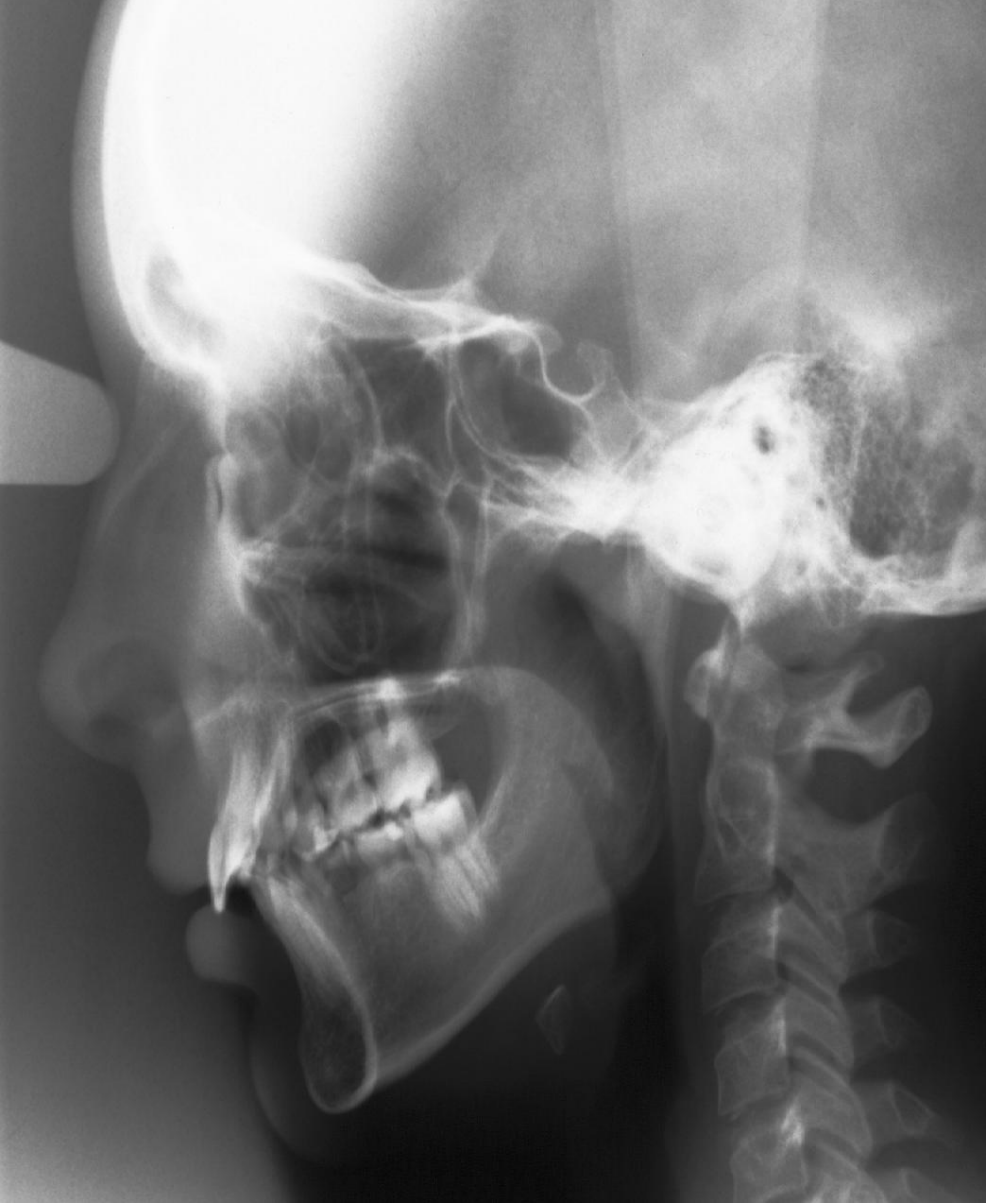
Radiographs of a person with 13 congenital missing teeth: lateral encephalometric radiograph.
